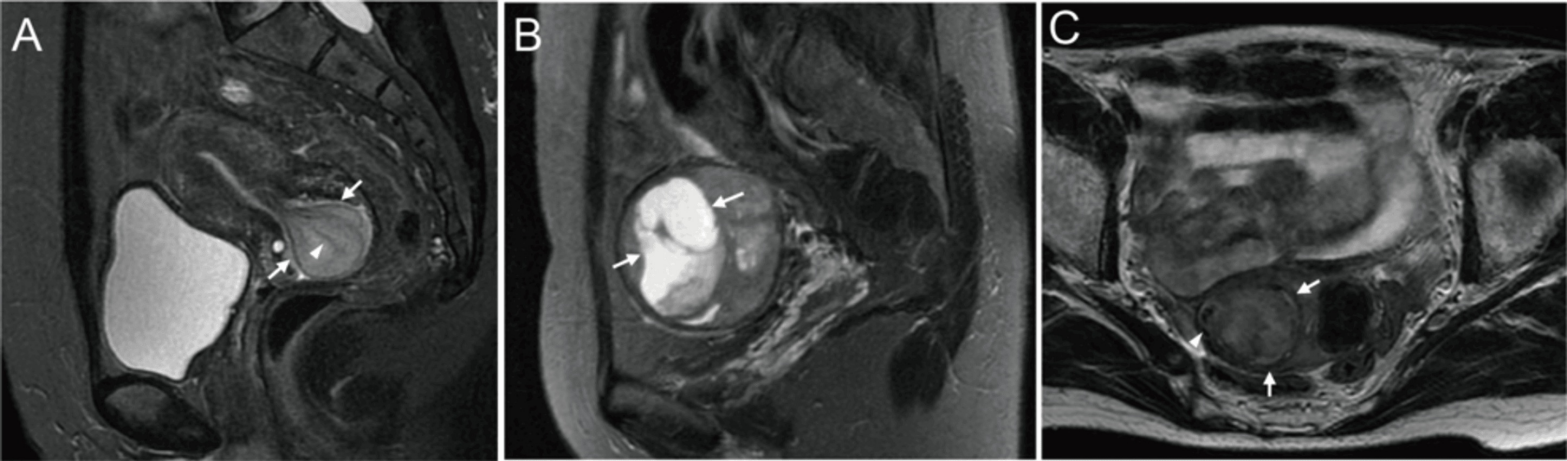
Magnetic resonance imaging findings in LGESS in representative cases A submucosal lesion is evident on sagittal T2WI (A) and protrudes into the vaginal canal (arrows); notably, an intramass band-like structure with low signal intensity (arrows) was observed (case 3). Sagittal T2WI (B) reveals an oval, well-defined, heterogeneously hyperintense myometrial mass with cystic areas (arrows; case 1). On axial T2WI (C), a T2 high-signal mass surrounded by a low-signal rim (arrows) exhibits nodular invasive growth (arrows; case 2). T2WI: T2-weighted imaging; LGESS: low-grade endometrial stromal sarcoma
